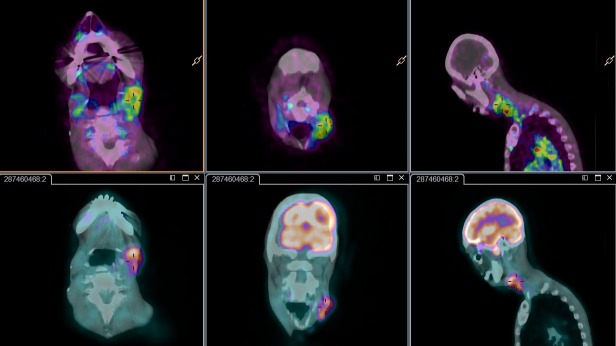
^89^Zr-RG7356 uptake in tumor lesions Representative images of lymph node metastases in left upper neck of a patient with advanced CD44-positive head and neck cancer. *Upper row*: ^89^Zr-RG7356 PET images acquired 5 days post injection. From left to right: axial, coronal and sagittal slices (8 mm) PET images fused with low-dose CT. Enhanced tracer uptake in malignant lymph nodes is shown (indicated by red center cross, SUV_max_ 3.9). *Lower row*: ^18^F-FDG PET images fused with low-dose CT (similar orientation as ^89^Zr-PET; SUV_max_ 7.8). Note that spatial distribution of ^18^F-FDG and ^89^Zr-RG7356 within the lesion is similar with central hypoactivity corresponding to necrosis.
